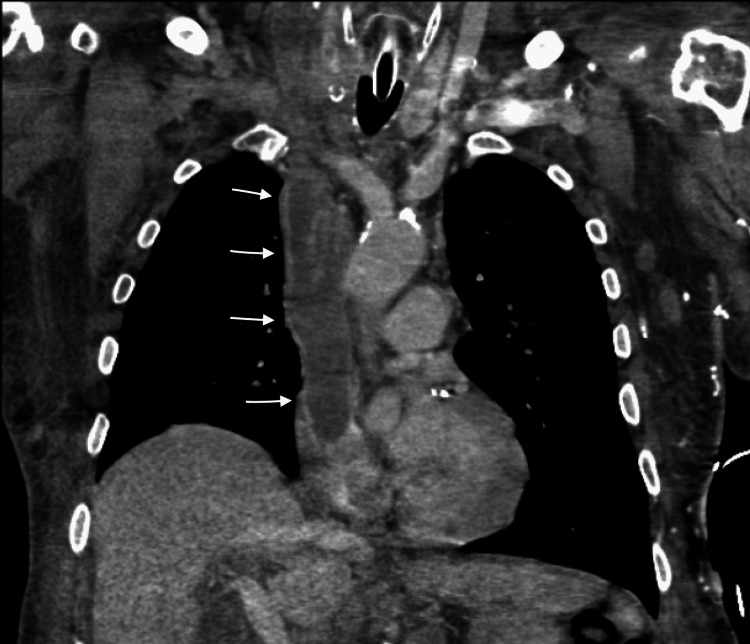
Coronal chest CT scan with arrows showing thrombus in SVC extending to right atrium
